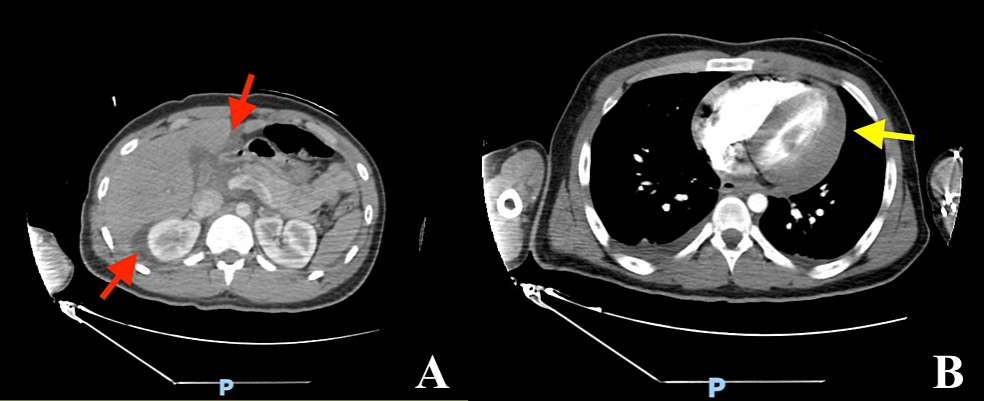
Axial Sections of Computed Tomography Scan of the Abdomen (A) and Chest (B) (A) Free fluid in the abdomen (red arrows). (B) Pericardial effusion (yellow arrow).
